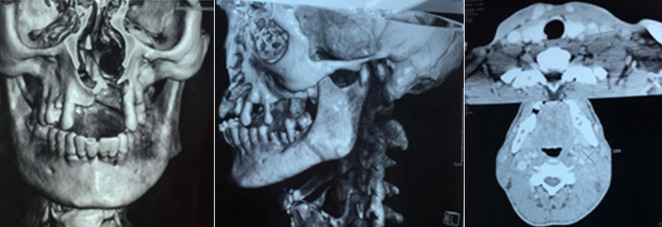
computed tomography scan of the facial mass showing a fistula between the left nasal cavity and hard palate
